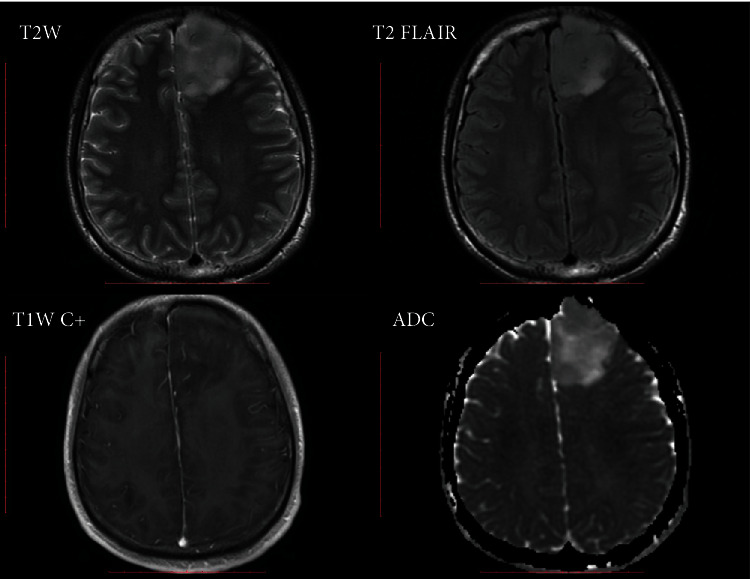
A 26-year-old man with an IDH1-mutant glioma (diffuse astrocytoma, WHO grade II). The tumor is located in the frontal lobe with no contrast enhancement, no deep white matter invasion, no necrosis, and an expansive tumor behavior (T1~FLAIR).
